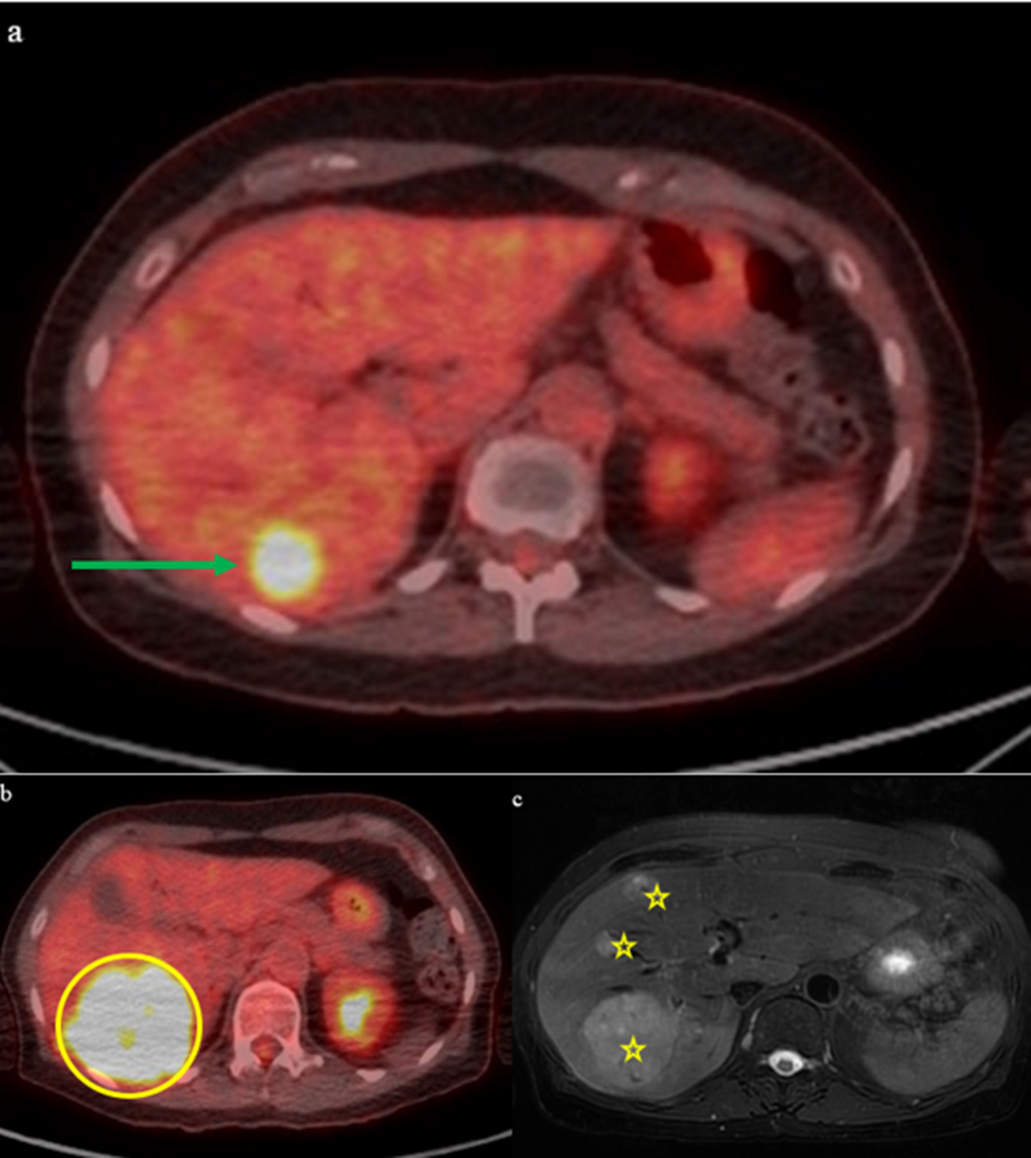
PET-CT scans identified new hypermetabolic nodes in the neck and a liver mass, both likely melanoma metastases. Follow-up PET-CT and MRI confirmed progression of hepatic metastases and raised suspicion of tumor foci in the abdominothoracic wall. (a) PET-CT on 8/31/2022 shows new mass in right lobe of liver measuring approximately 3 cm (green arrow). (b) Follow-up PET-CT in December 2022 shows interval progression of FDG-avid hepatic metastasis (yellow circle). (c) Associated follow-up MRI in December 2022 demonstrates multifocal hepatic metastatic disease progression (yellow stars).
